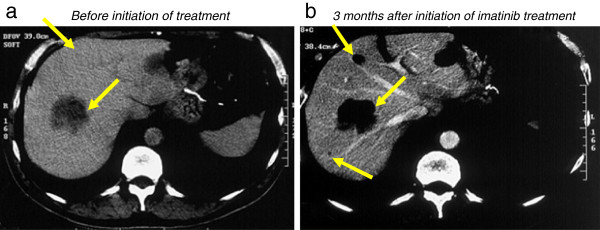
CT scans of the patient before and after imatinib treatment for metastatic GIST. Tumor masses (arrow) can be seen in the liver of the patient before initiation of imatinib treatment (a). The masses (arrow) became hypodense and homogenous on CT obtained 3 months after imatinib treatment (b), indicating good response to imatinib.
